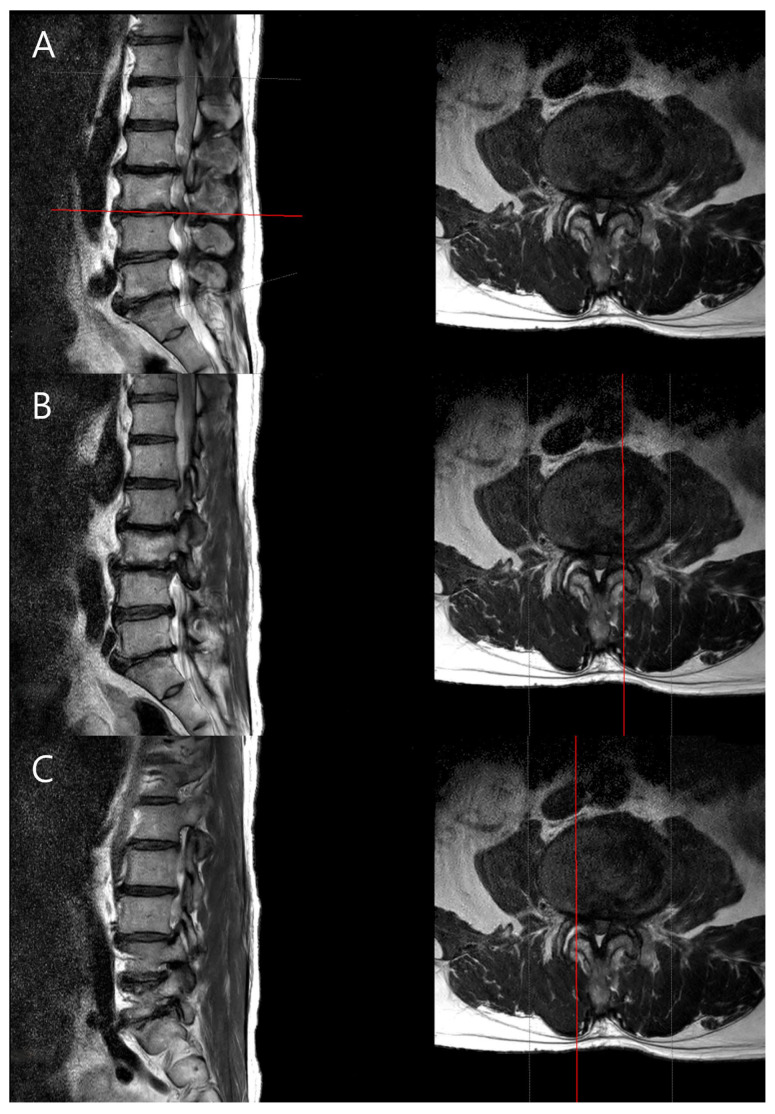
Evaluation of lumbar disc degeneration using T2 MRI images. (A) Axial plane showing the disc level. The horizontal red line on the sagittal image indicates the level of the axial cut shown on the right. (B,C) Sagittal and axial images were used to assess differences in disc degeneration between the concave and convex sides. The vertical red lines on the axial images delineate the sagittal cut plane, specifying whether it represents the concave or convex side for assessment.
